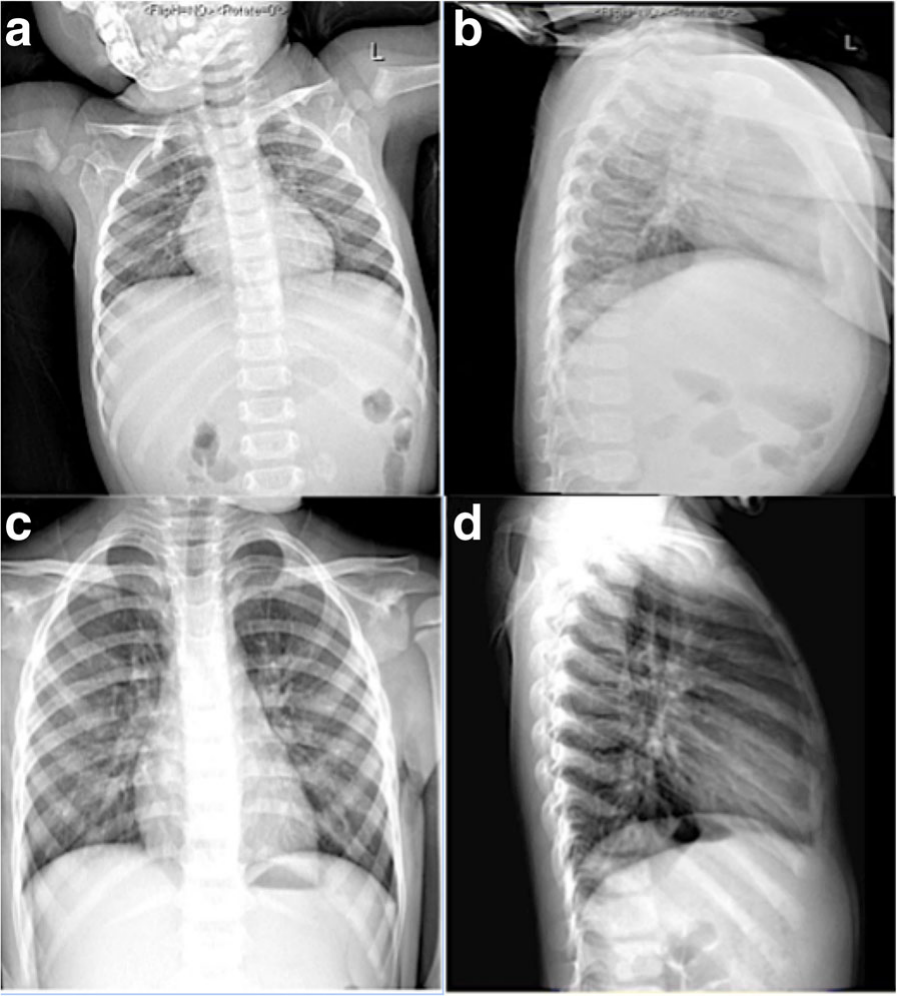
a At our patient’s first visit (8-months old), chest radiography showed narrowing of aortic knob and enlargement of right atrial at the frontal position. b At the same time, pulmonary blood stasis and increase of lung markings were also found at the lateral position, those image features may have been caused by atrial septal defect. c When he was 3-years old, narrowing of aortic knob and enlargement of right atrial were improved slightly compared with his first visit. d However, at the lateral position, increase of lung markings and pulmonary blood stasis were obviously aggravated compared with his first visit, those image features were attributed to the deterioration of atrial septal defect
